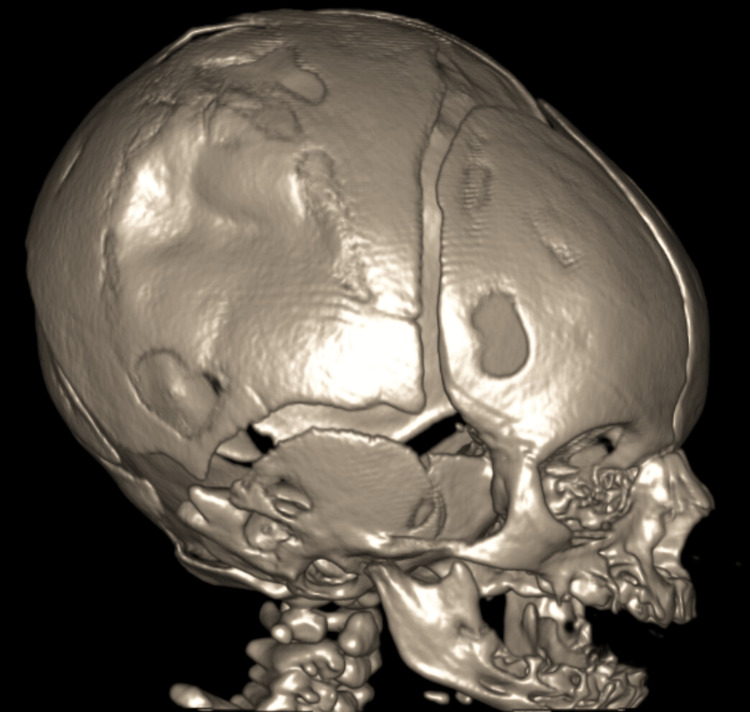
CT head with skull reconstruction showing right parietal bone depression with no visualized fracture lucency or acute hemorrhage, with intact gray-white matter differentiation without mass effect or midline shift.
